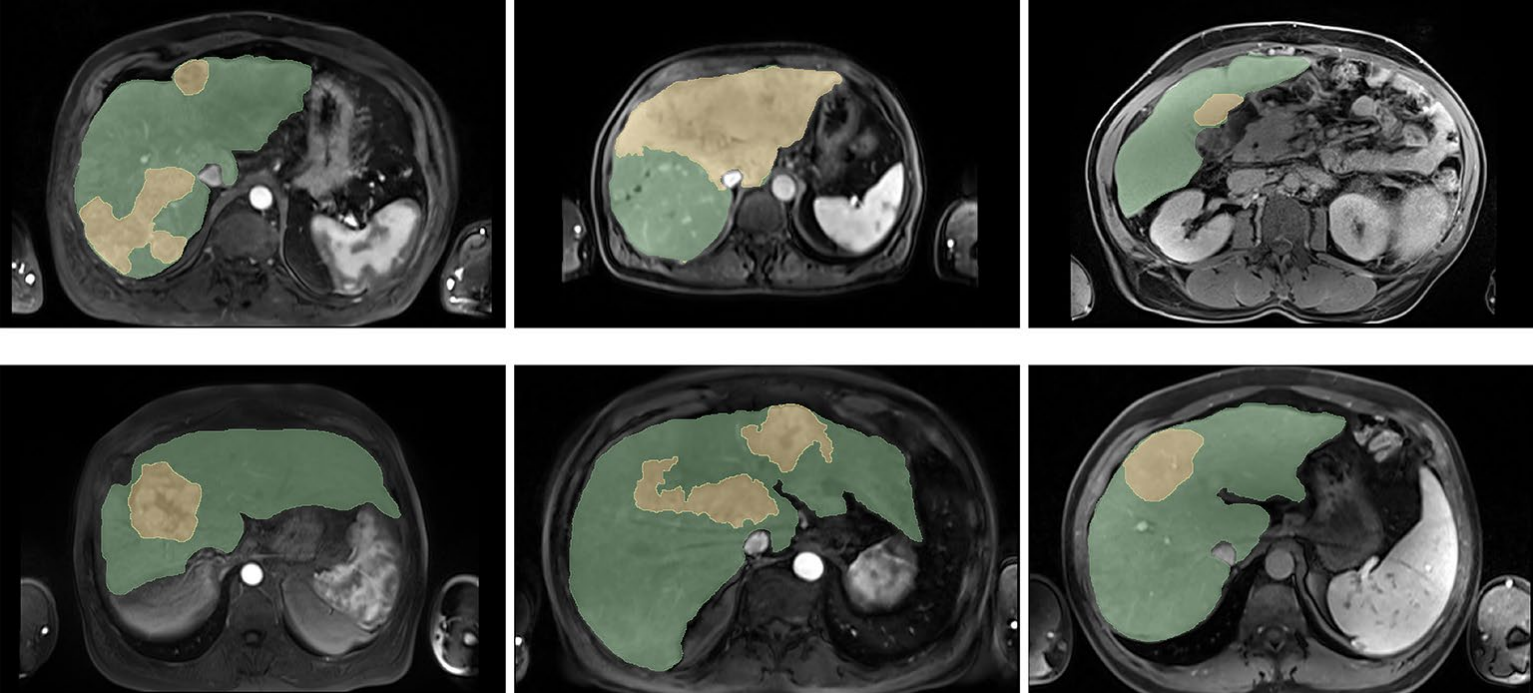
Axial slices of six contrast-enhanced magnetic resonance images from six different patients with the corresponding image labels superimposed. The liver appears in green and tumour in yellow.
